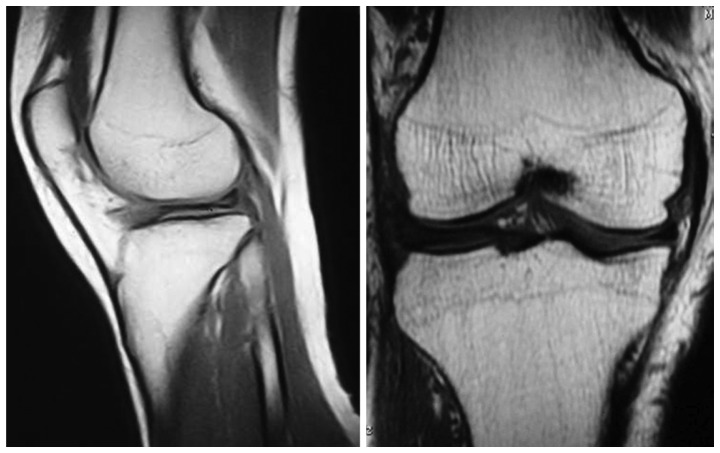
MRI shows injured discoid lateral meniscus.
